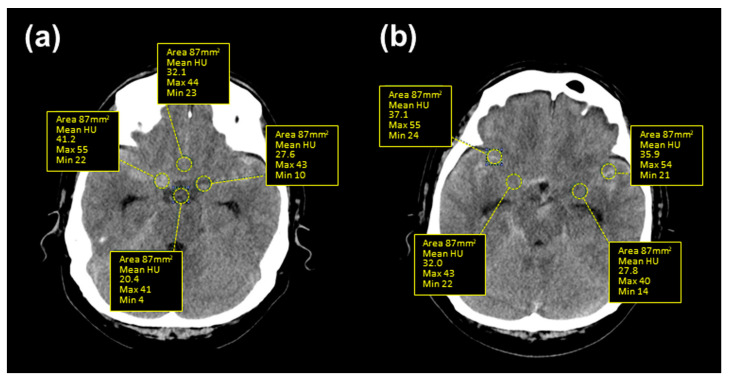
Representative brain CT imaging showing the location of analyzed HU values for assessing the density of the SAH clot. The HU values of the basal cistern (a) and Sylvian cistern (b) were measured as the average of the circular ROI values (87 mm^2^) of the bilateral carotid, interpeduncular, and lamina cisterns (a) and bilateral medial and lateral Sylvian cisterns (b), respectively. Each value has been shown to predict symptomatic vasospasm and hydrocephalus in patients with mild-grade SAH treated with endovascular coiling [16].
